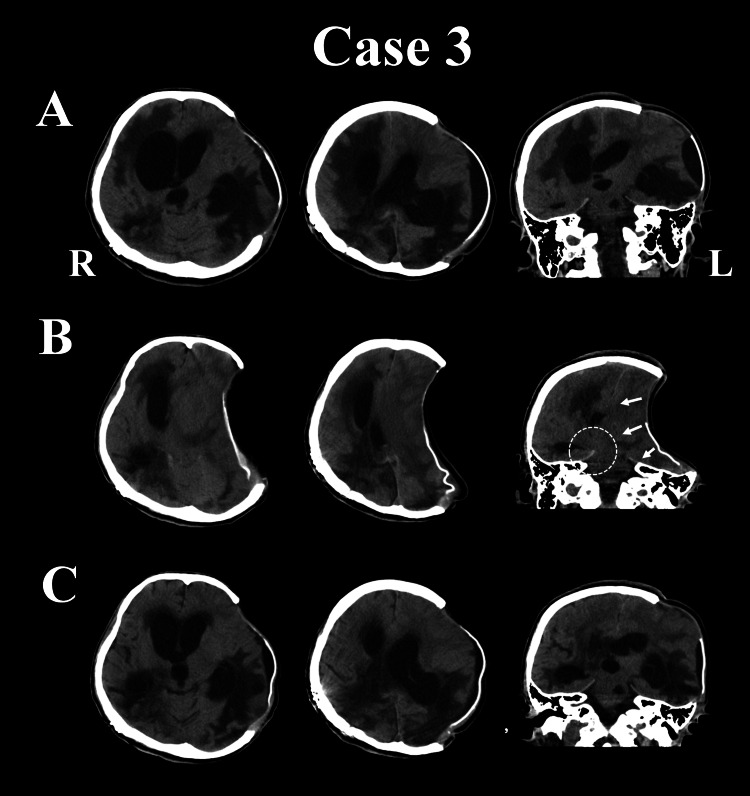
CT findings in Case 3 (A) The lateral ventricles are tremendously enlarged, especially on the right side, with obvious periventricular lucency. Additionally, the skin at the site of the decompressive craniectomy is bulging, forming a thin subdural hygroma. (B) There is severe skin sinking at the site of the bone defect, and the left ventricle is difficult to recognize because of the compression. The midline has shifted significantly to the side contralateral to the bone defect, suggesting impending brain herniation with the Kernohan notch phenomenon (white circle) caused by the inward and downward compression (white arrows). (C) The midline shift has improved, and the periventricular lucency at the right anterior horn has resolved. The sulci and ambient cistern have become clear, and the pressure on the midbrain has also eased. CT: computed tomography
